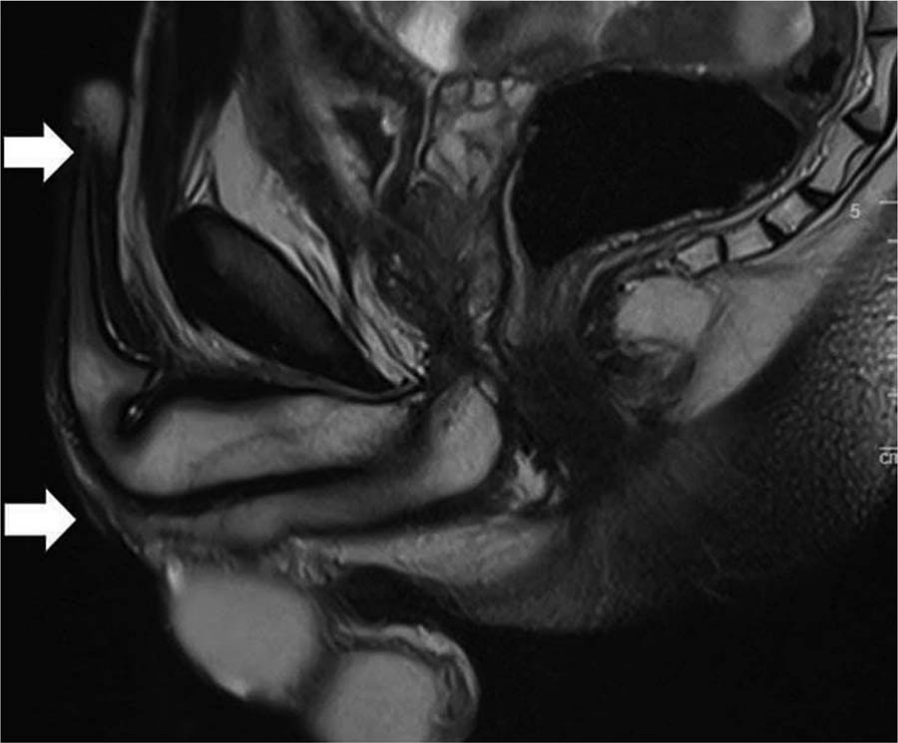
Sagittal MRI of the pelvis demonstrating a pan-urethral stricture. The urethra shows a long segment narrowing extending between the two arrows.
